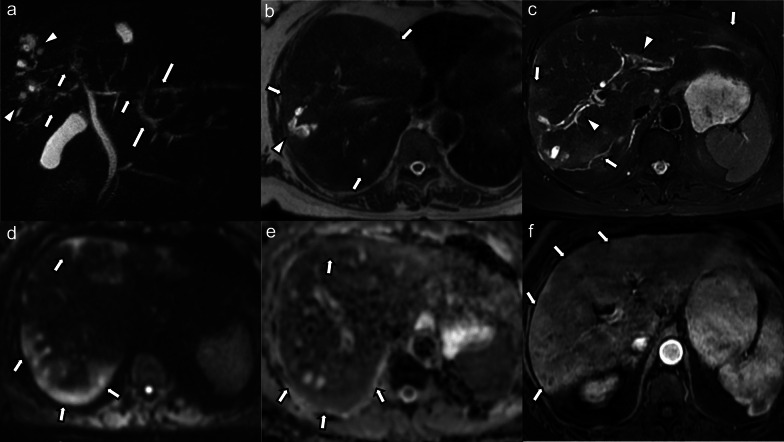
69-year-old male patient with COVID-19-associated SSC complicated by acute bouts of cholangitis with development of multiple biliary abscesses requiring prolonged antibiotic and interventional therapy. a Maximum intensity projection MRCP image shows multifocal areas of bile duct stricturing (arrows) in both liver lobes with intermittent mild upstream dilatation (long arrows). In the right liver lobe, there are clustered cystic changes representing saccular dilatation of bile ducts (arrowheads). b Axial non-fat-suppressed and (c) fat-suppressed T2-weighted images show multiple patchy hyperintense parenchymal changes in the periphery and subcapsular liver (arrows in b and c). Saccular dilatation of peripheral bile ducts (arrowhead in b) with intraluminal debris. There are hyperintense peribiliary signal changes (arrowheads in c). d High *b*-value diffusion-weighted images and (e) corresponding ADC map again show these peripheral and subcapsular signal changes with mild diffusion restriction (arrows). f On post-contrast arterial phase images these parenchymal changes are hyperenhancing (arrows)
